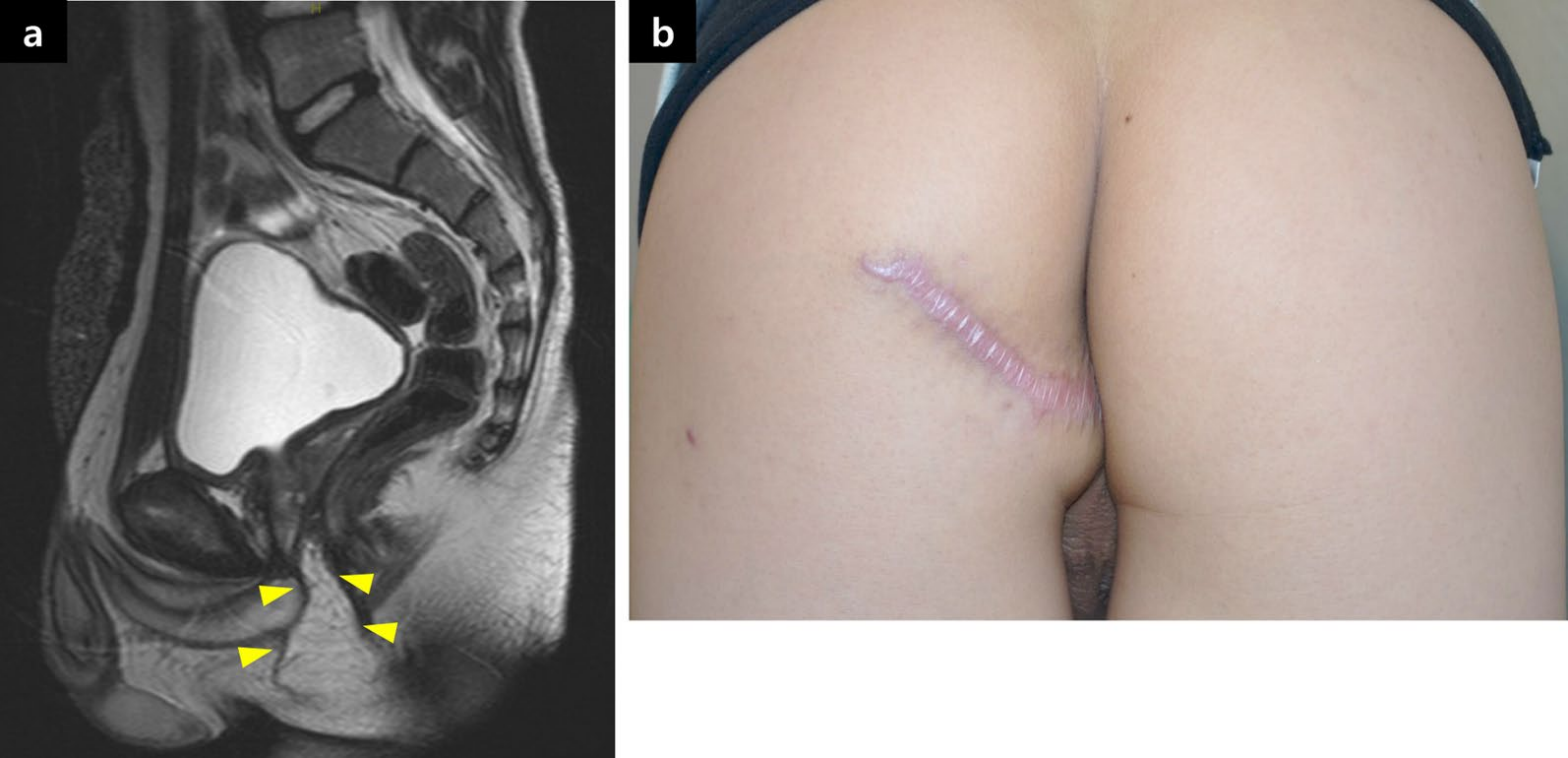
Postoperative images. a Pelvic magnetic resonance imaging 5 months postoperatively revealed the interposed flap between the urethra and rectum without atrophy and deviation (yellow arrowhead). b Scars on the buttock
